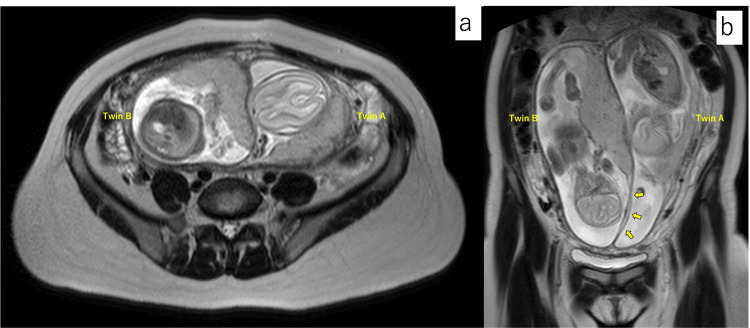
MRI T2-weighted images at 20 weeks of pregnancy. (a) Axial image showed the placenta of Twin B attached to the septum. (b) Coronal image showed the thin septum without placenta of Twin B (yellow arrows). MRI: magnetic resonance imaging
